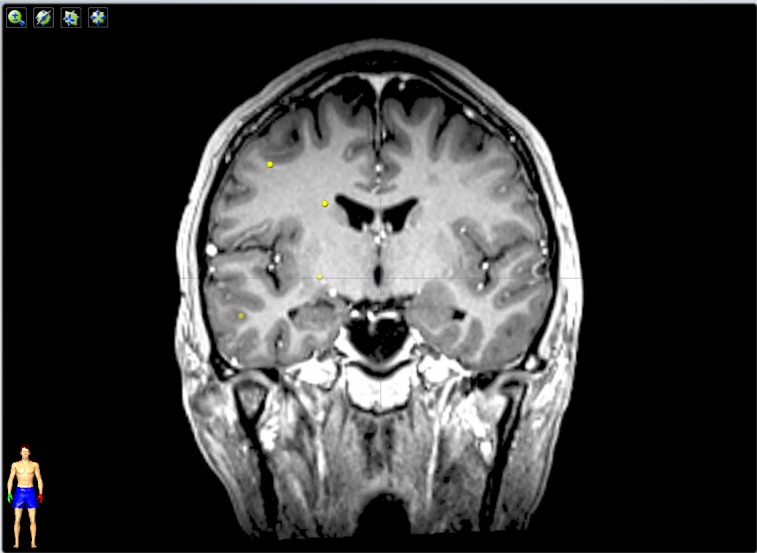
Post-SRS MRI scan The latest MRI of the brain shows complete resolution and local control of the treated lesions.
